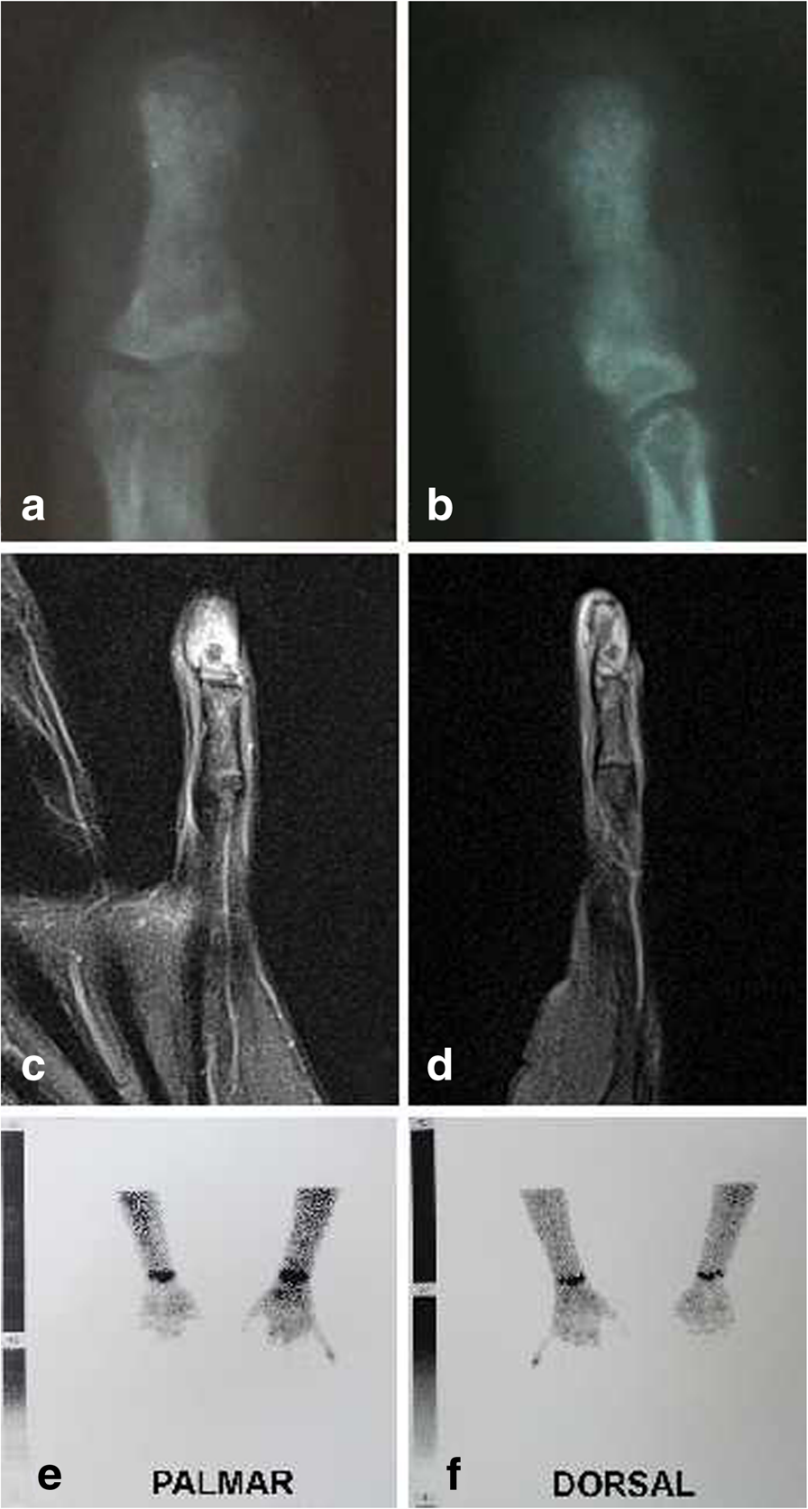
A follow up radiographs of the left small finger distal phalanx in anteroposterior (a) and lateral (b) view obtained two years after the symptoms begun. Diaphyseal distal phalanx widening and a central radiolucent zone are observed. Magnetic resonance image of the left small finger in proton density fast spin echo sequence in coronal (c) and sagittal (d) plane. Signal hyperintensity in the area of the distal phalanx that correlate with soft-tissue oedema is observed. On the dorsal aspect of the affected phalanx, a hypointensive oval lesion with no signs of cortical destruction is observed. These findings resemble an osteoid osteoma or sequestrum. An image of hands radionuclide skeletal scintigraphy with Technetium 99 m-MDP report in two projections palmar (e) and dorsal (f). Increased radionuclide uptake in distal phalanx/interphalangeal joint of the affected finger is predominantly observed in the dorsal projection
